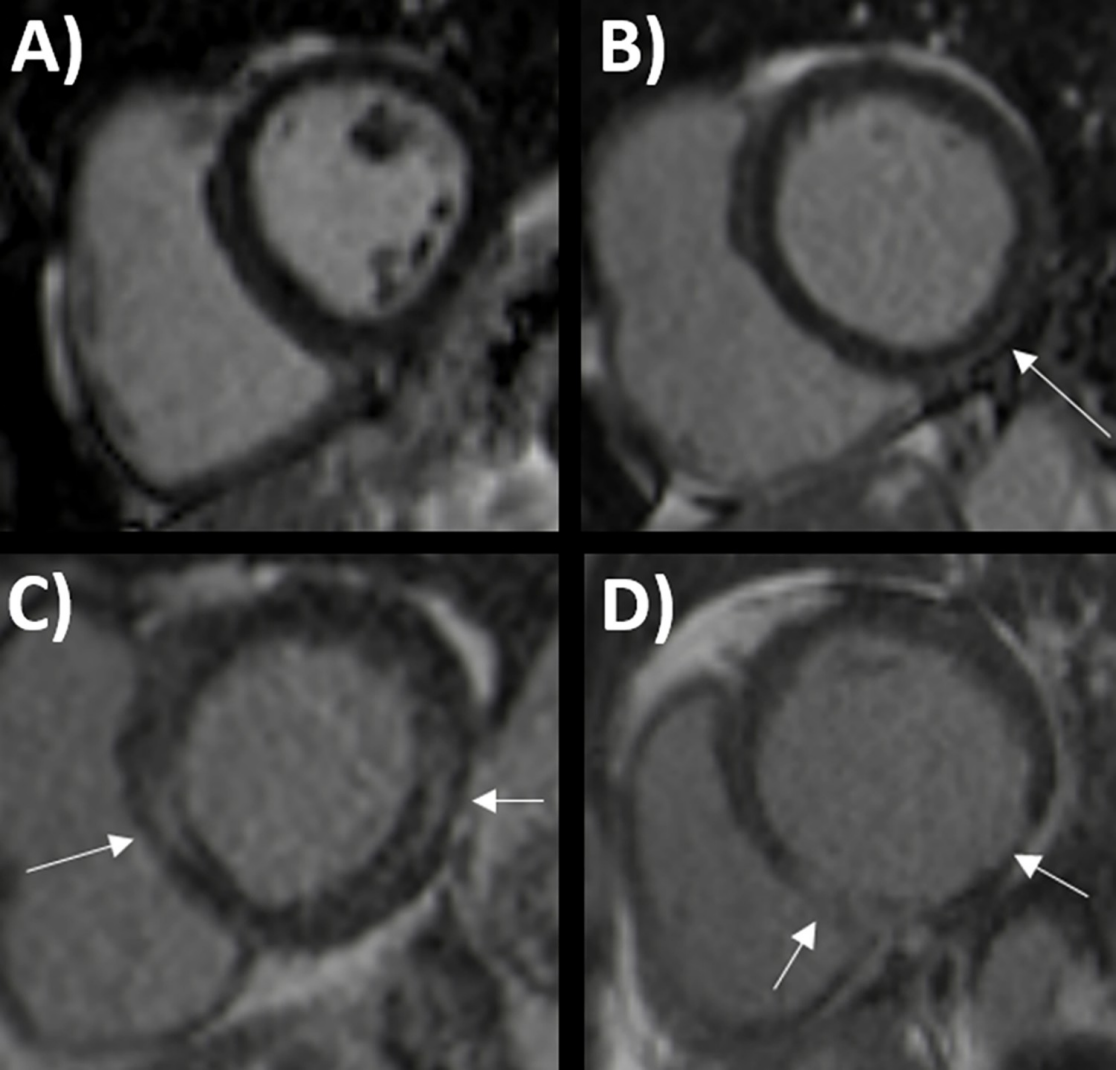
Examples of cardiac magnetic resonance imaging findings in patients with coronavirus disease 2019 (COVID-19). Normal left ventricle (LV) without late gadolinium enhancement (LGE) (A). Subepicardial LGE in inferolateral LV wall suggestive of previous myocarditis (B). More severe LGE with a septal and inferolateral non-ischemic pattern concordant with previous myocarditis (C). The inferior wall has extensive subendocardial and transmural scarring due to previous myocardial infarction (D). White arrow = LGE.
